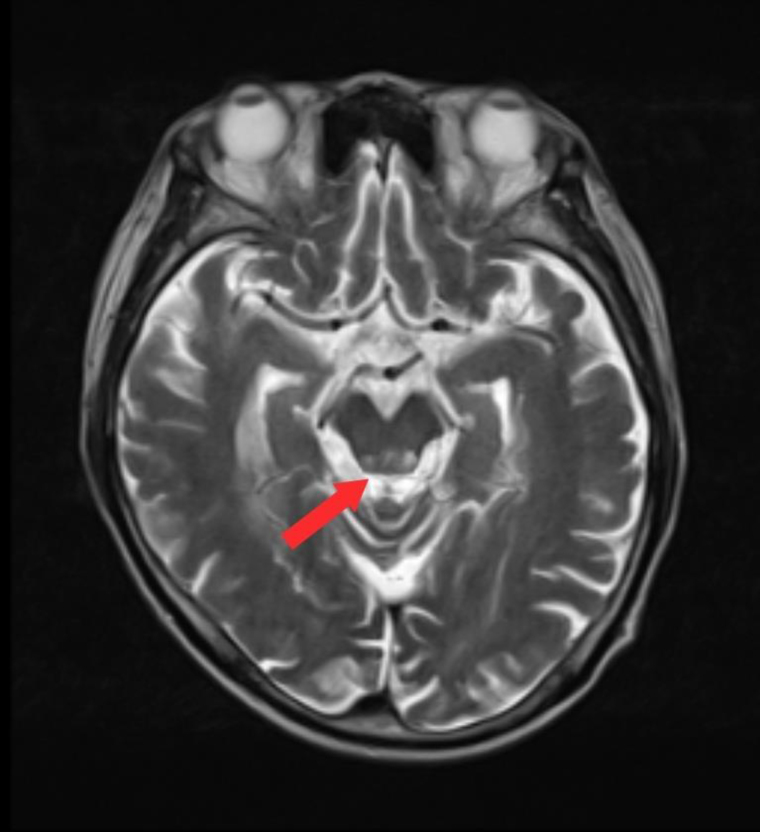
Magnetic resonance imaging manifestation reveals abnormal symmetric signals in the pericentric region of the midbrain, symmetrically distributed patches with slightly prolonged T1 and T2 signals around the bilateral lateral ventricles.
